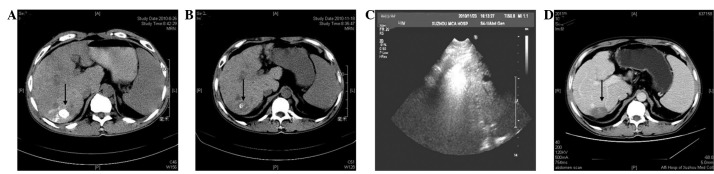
Diagnosis images of patient 1 at follow-up. (A) Axial non-contrast CT image obtained one week after TACE reveals complete iodized oil retention inside the HCC (arrow). (B) Axial non-contrast CT image obtained 3 months after TACE reveals the almost complete disappearance of iodized oil inside the HCC (arrow). (C) Sonogram obtained during sonographically-guided percutaneous microwave ablation. (D) Axial contrast-enhanced CT scan obtained 9 months after microwave ablation reveals an area of hypoattenuation without enhancement, indicative of a complete response (arrow). TACE, transcatheter arterial chemoembolization; CT, computed tomography; HCC, hepatocellular carcinoma.
